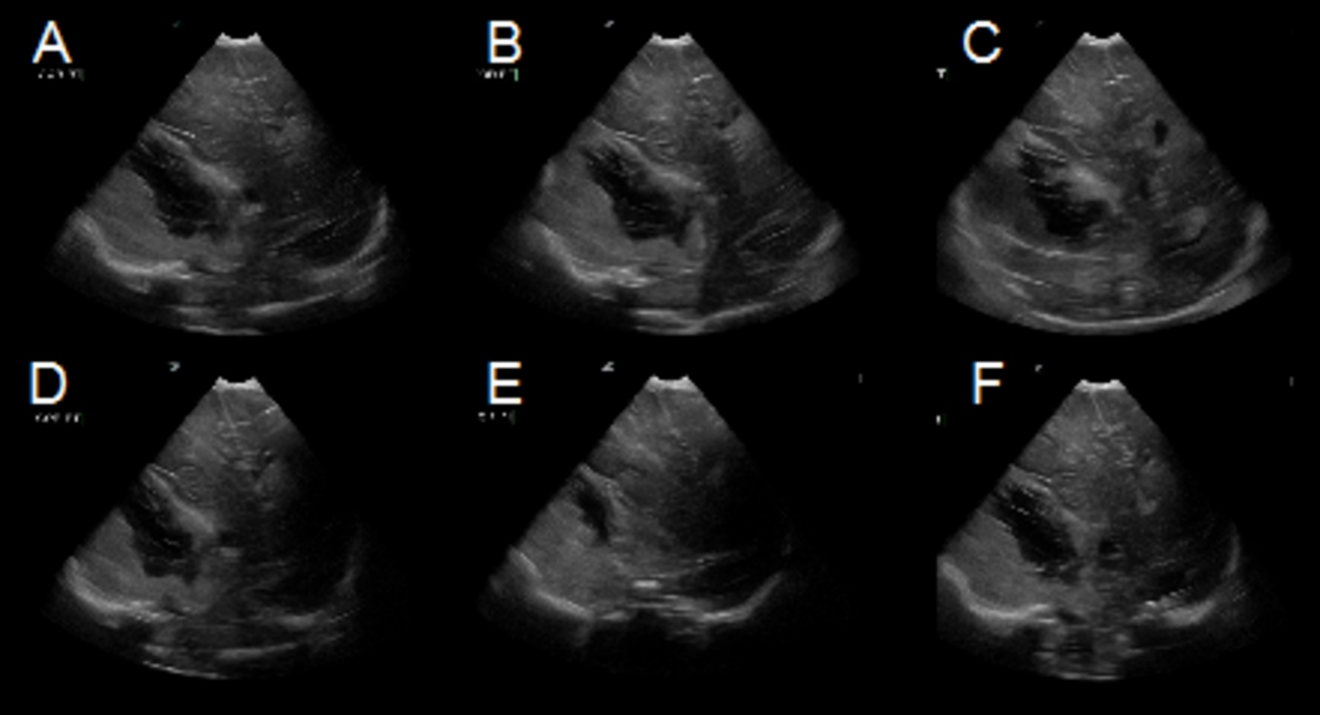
Coronal views of head ultrasound on Day of Life 2. Coronal views from posterior (A) to anterior (F) showing a large right-sided extra-axial hematoma.
